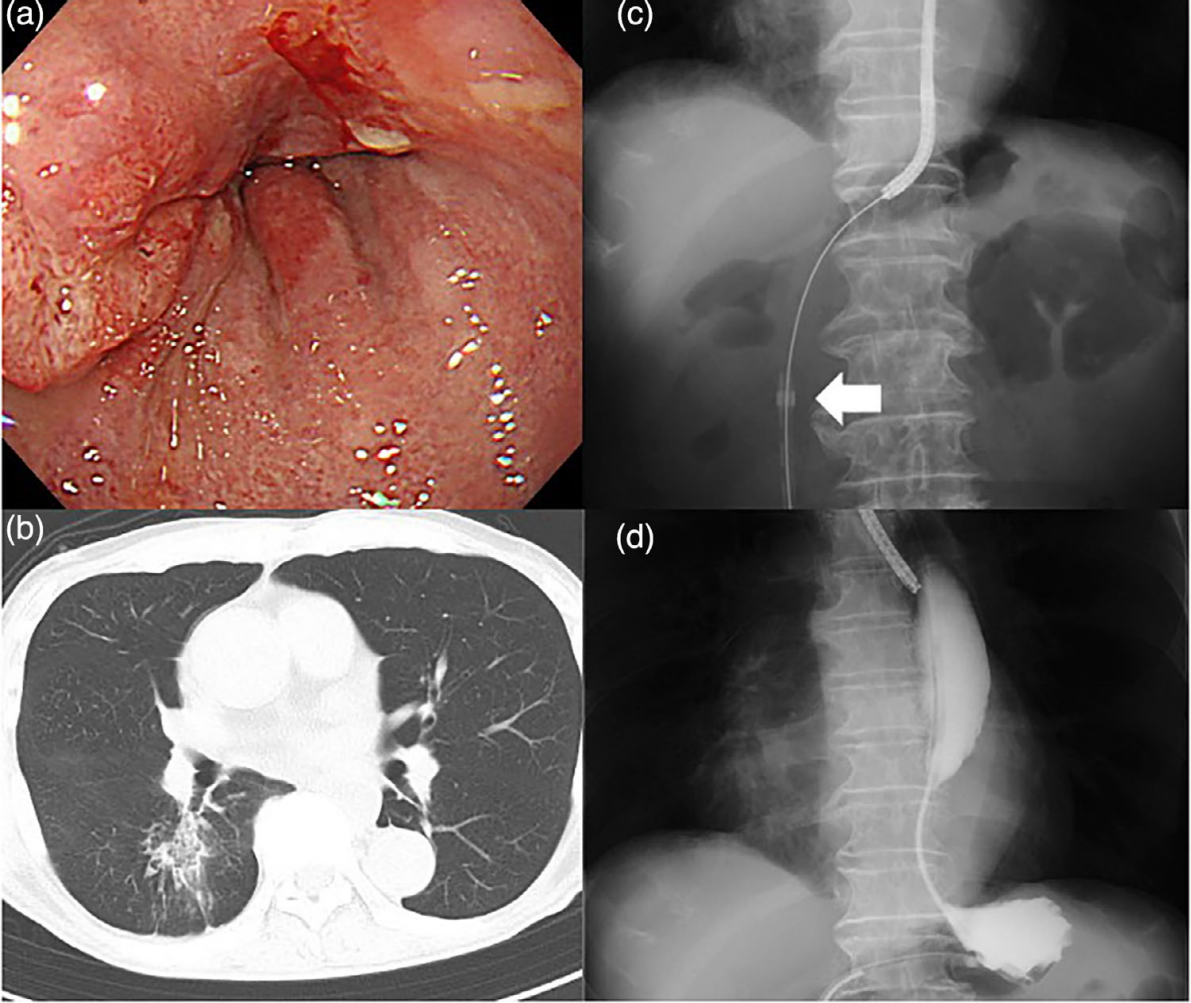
The insertion of percutaneous endoscopic transgastric jejunostomy retrogradely in Case 2. (a) Endoscopy and computed tomography findings of the tumor with the stricture. (b) The pooling of saliva caused aspiration pneumonia prior to management. (c) An ultrathin endoscope is passed through the stricture, and a percutaneous endoscopic transgastric jejunostomy catheter is inserted retrogradely from the gastrostomy stoma under the guidewire. The white arrow indicates the tip of the PEG‐J catheter. (d) Contrast test with Gastrografin shows the dilatation of the esophagus and the malignant stricture after catheter insertion.
